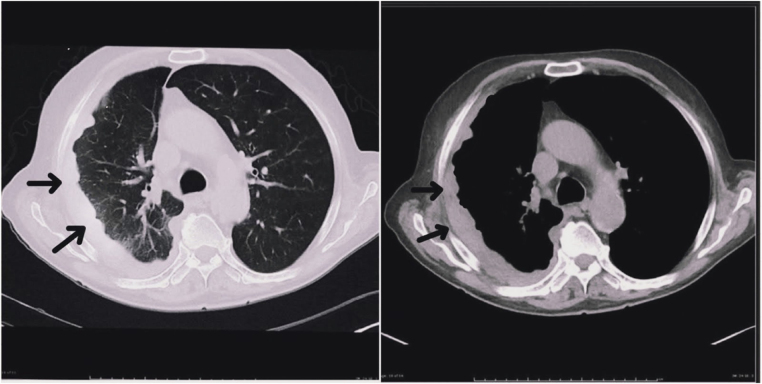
High-resolution and contrast-enhanced computed tomography of the chest showing diffuse nodular and rind-like thickening of the right pleura with smooth undulating margins (black arrows), minimal residual pleural fluid, and underlying lung compression, characteristic of malignant pleural mesothelioma.
